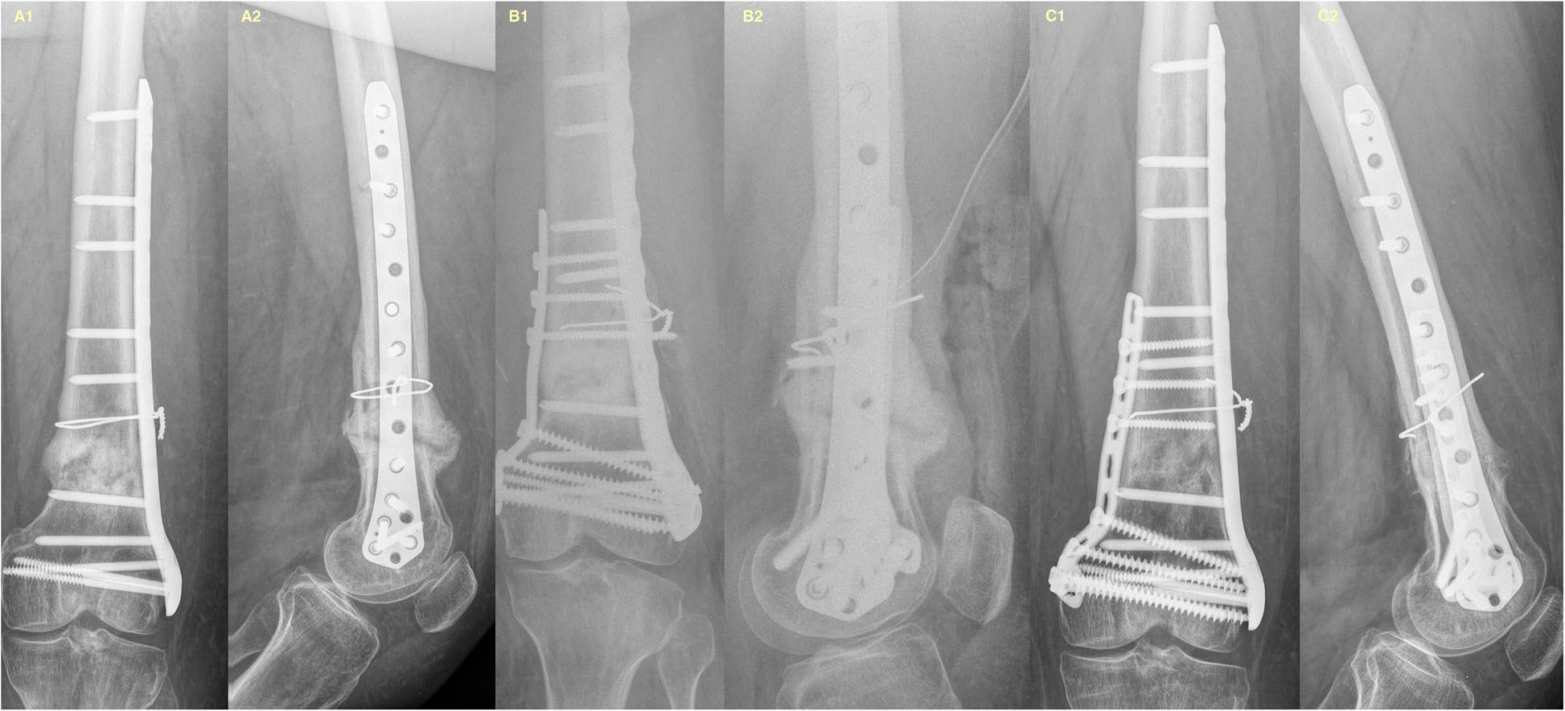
A Initial AP and lateral radiographs from a 24-year-old male with a BMI of 40.36 who was referred with hypertrophic nonunion with a stable in situ locking plate construct. B Postoperative X-rays after revision with complete debridement of the nonunion site, bone grafting and medial augmentation plating with a locking plate. C The patients’ final AP and lateral X-rays showing bony healing of the nonunion
